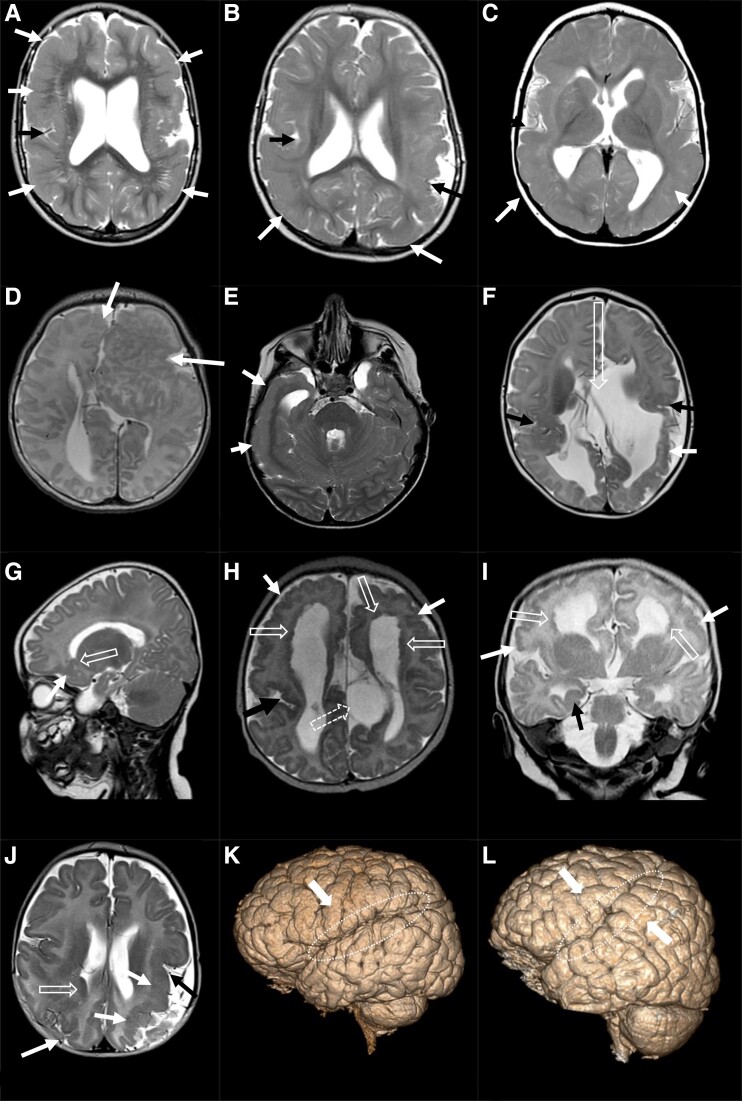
MRI features. (A–C) Axial T2 of PMG (solid arrows) with peri-sylvian distribution in three individuals with CMV infection. Black arrows for sylvian fissure. White arrows for PMG. (A) Bilateral peri-sylvian distribution, more severe left then right, with bilateral frontal and less parietal extension with effacement of sulci. Left sylvian fissure opened due to more extensive PMG; (B) bilateral peri-sylvian with predominantly posterior extension with more marked extension in the left than the right parietal lobe causing widening of left subarachnoid space; (C) with parietal and occipital distribution, arrows on superior occipital gyri. (D–F) Axial T2 of PMG after probable pre-natal vascular insult. (D) Left frontal dysgyria and PMG with a deeply infolded heterotopic grey matter brain malformation in the anterior left frontal lobe with anomalous subarachnoid spaces and vessels (not present on the image) with focal PMG also in the right frontal lobe (GS performed, unknown aetiology); (E) right temporal PMG with white matter volume loss and dilated temporal horn of the lateral ventricle (*NPHP1*); (F) bilateral peri-sylvian PMG (black arrows) with parasagittal volume defect (open arrow) and posterior white matter volume loss with PMG in the left inferior parietal lobe (genetic evaluation not performed). (G–J) T2 of sub-ependymal heterotopias (open arrow) and overlying PMG (white arrows). (G) Sagittal T2 with sub-ependymal heterotopia in left frontal horn with PMG in orbital gyri (*PTPN23*); (H) axial T2 with sub-ependymal heterotopia in bilateral frontal horns of lateral ventricles with PMG in frontal lobes. Right-sided peri-sylvian PMG (black arrow) and left parietal parasagittal cyst displacing the left thalamus inferiorly (dotted opened arrow); (I) Cor T2 of the same patient as in H with right-sided incomplete hippocampal inversion (globular shape, black arrow). Bilateral PMG (white arrows) with sub-ependymal heterotopia (open arrows) (Aicardi syndrome, GS trio with normal results); (J) sub-ependymal heterotopia in right trigonal part of lateral ventricles (open arrow on right side) with PMG in right parietal (white arrow). Left peri-sylvian PMG (black arrow) with left frontal and parietal distribution (white arrows) (parents declined genetic evaluation, CMV excluded). (K and L) 3D volume of right hemisphere with sylvian fissure (dotted circle). (K) Posterior ascending ramus of sylvian fissure extending horizontally into inferior parietal lobe with frontal PMG (white arrow) (*PIK3CA*); (L) horizontal ramus projects steep into ascending ramus of the sylvian fissure with a near vertical extension into the superior parietal lobe with peri-sylvian and peri-opercular PMG (white arrows) (GS performed, unknown aetiology).
